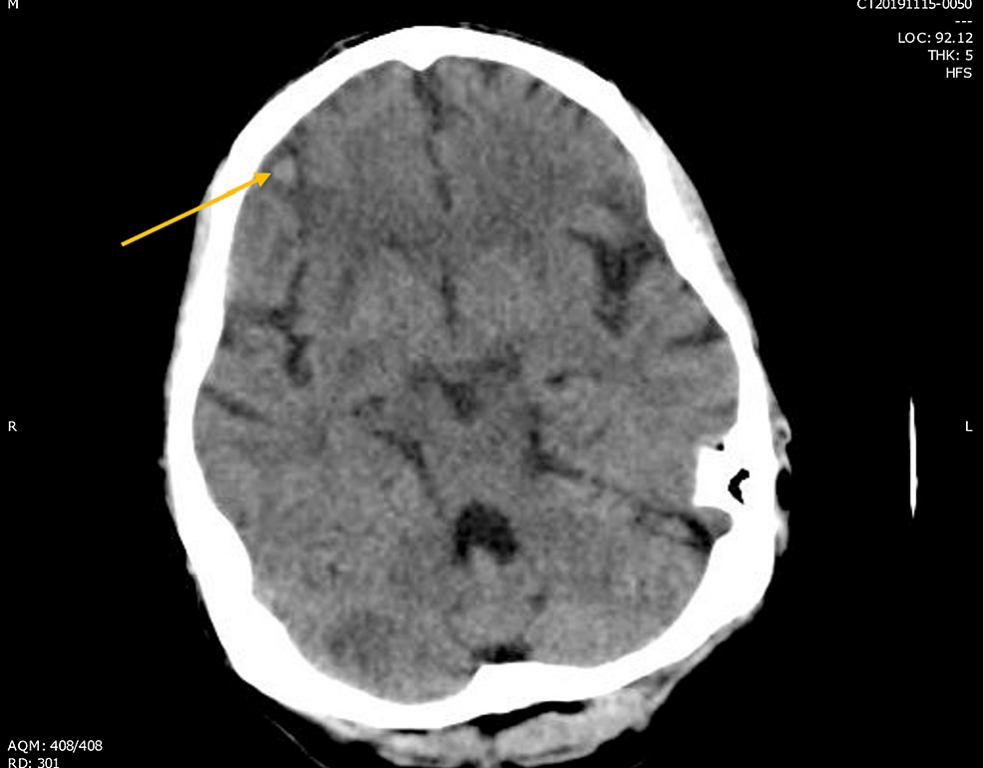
Arrow showing suspected cerebral hemorrhage on CT head.
